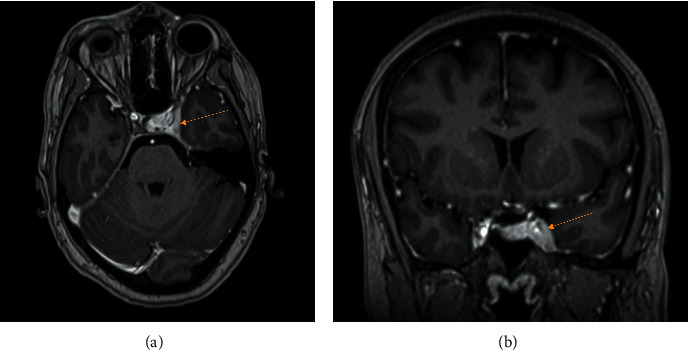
T1-weighted postcontrast MRI brain showing abnormal enhancement of the left cavernous sinus (arrows) in the axial and coronal views, respectively.
